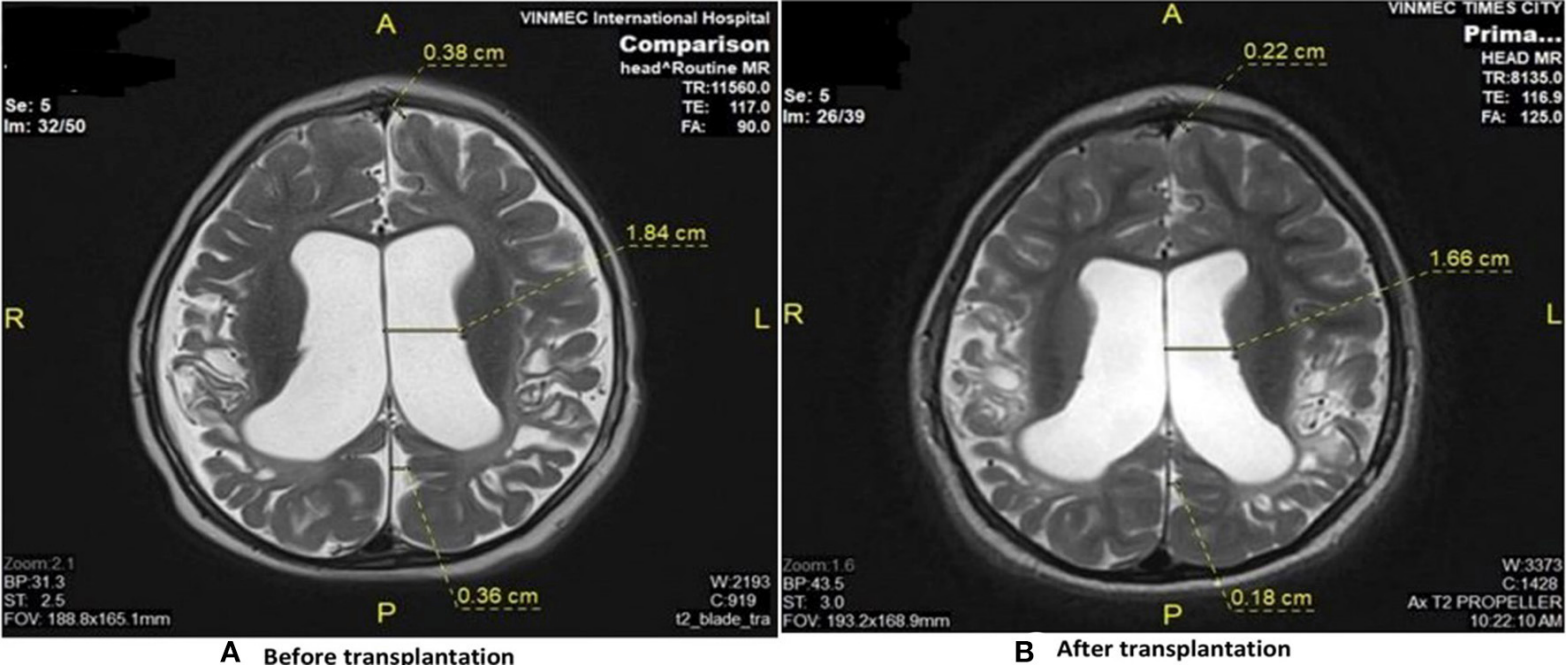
Brain MRI results revealed the improvement before and after stem cell transplantation. (A) Diffuse cortical and subcortical lesions with diffuse brain atrophy and dilated ventricles before transplantation. (B) Diffuse brain atrophy, and dilation of ventricles reduced after transplantation.
